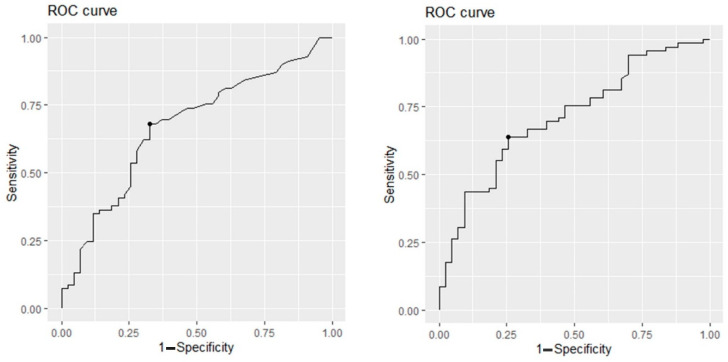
Receiver operating characteristic (ROC) curves for NSD identification according to pineal body SUVmax (left, AUC = 0.67) and pineal to occipital cortex uptake ratio (POR, right, AUC = 0.71).
